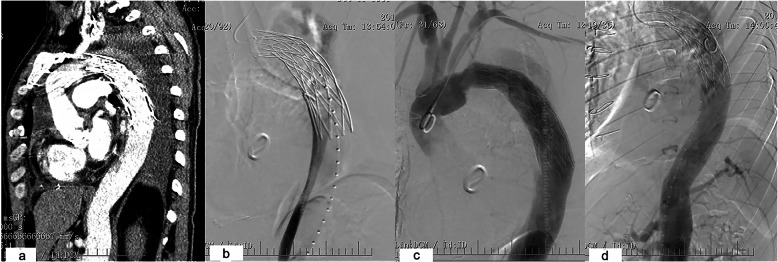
a The elephant trunk was found in the wrong place based on CT. b In the angiography of the femoral artery, the true lumen was constricted and very small. c Trunk in the false lumen. d The blood supply of abdominal organs was from the false lumen
